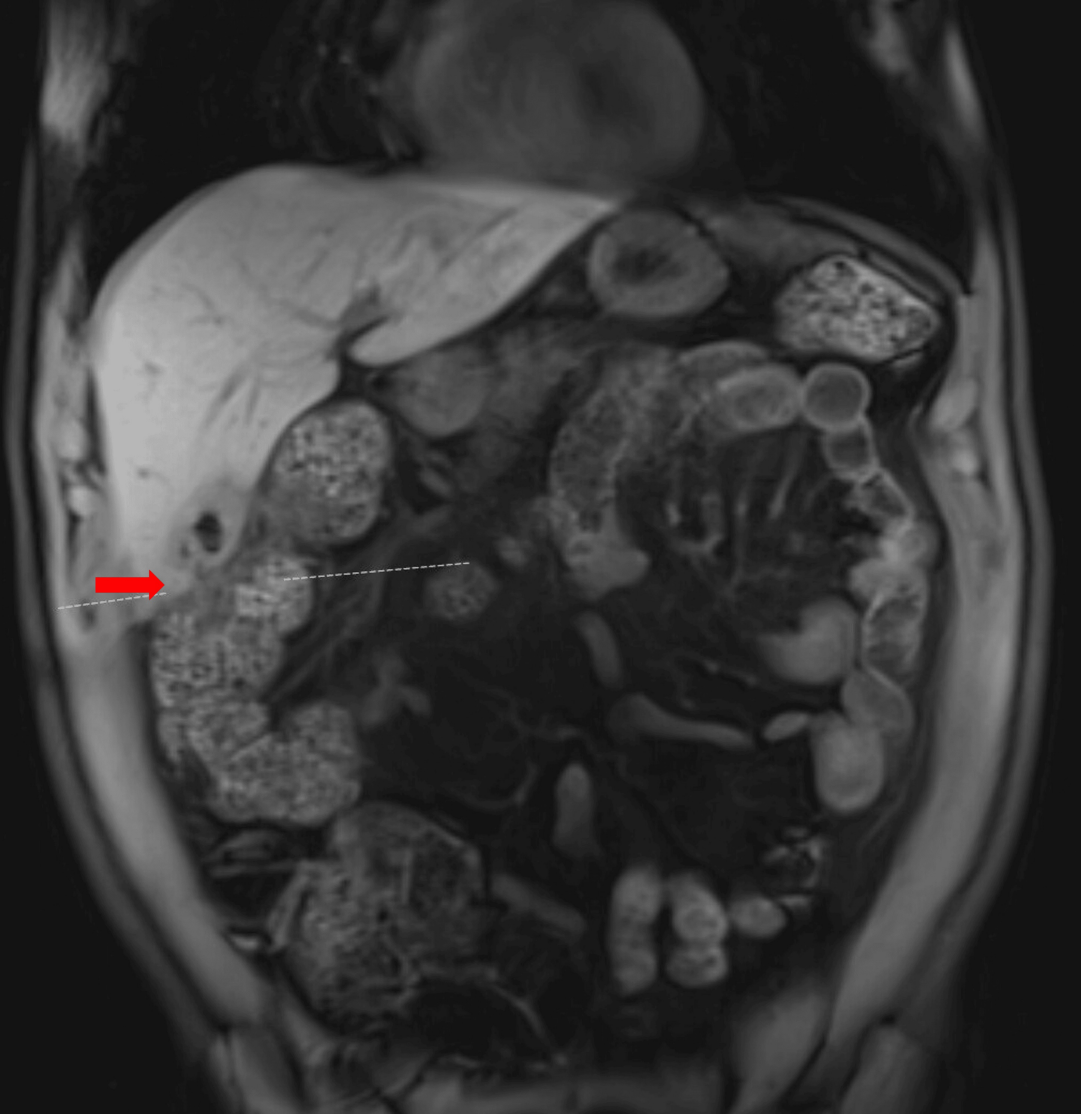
MRI of the gallbladder showing a cholecystocolonic fistula at the hepatic flexure. The gallbladder was thick-walled and hyperemic. Red arrow: cholecystocolonic fistula. MRI, magnetic resonance imaging;
